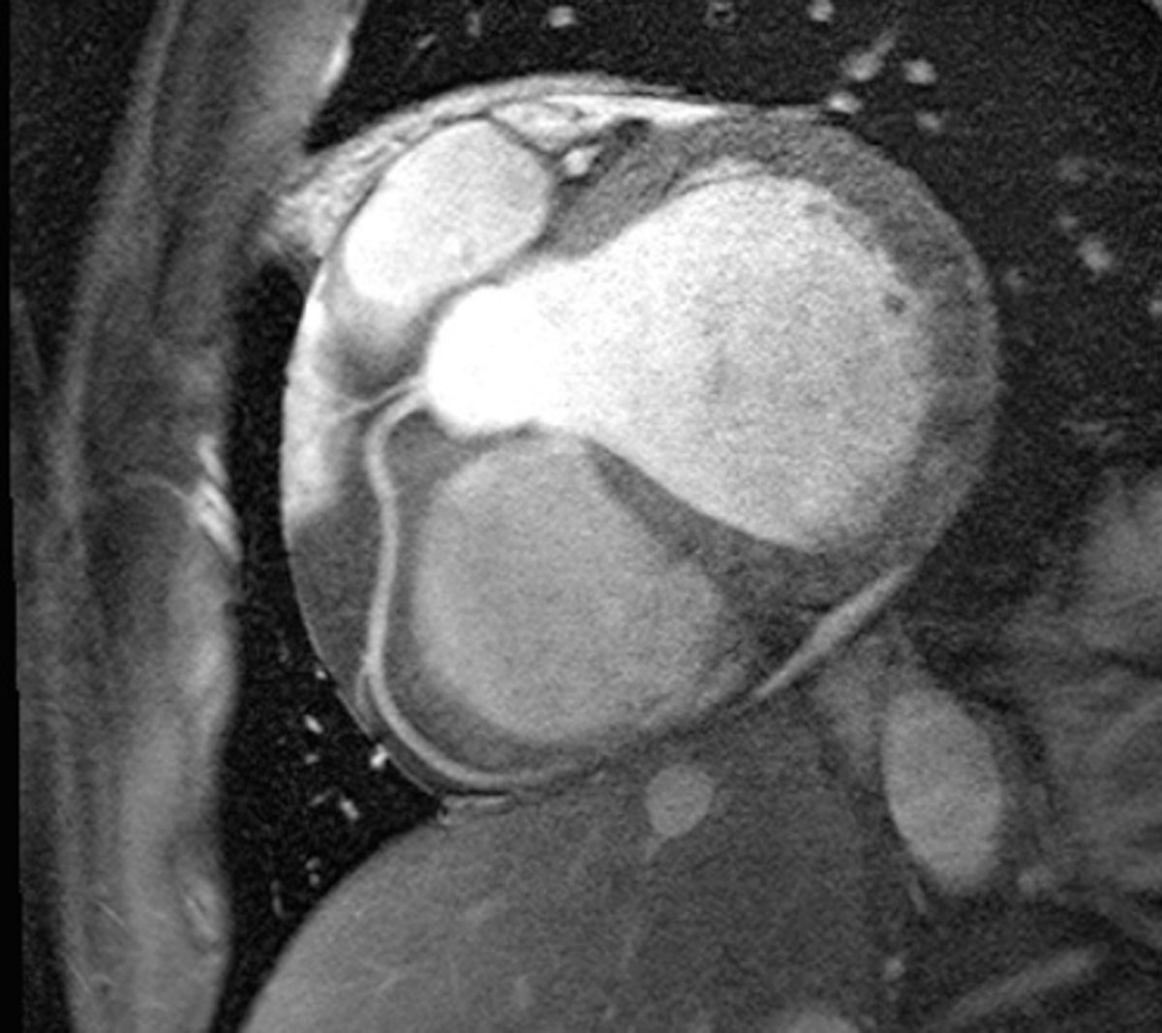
# Value of a hybrid PET/MRI in the assessment of cardiac viability
